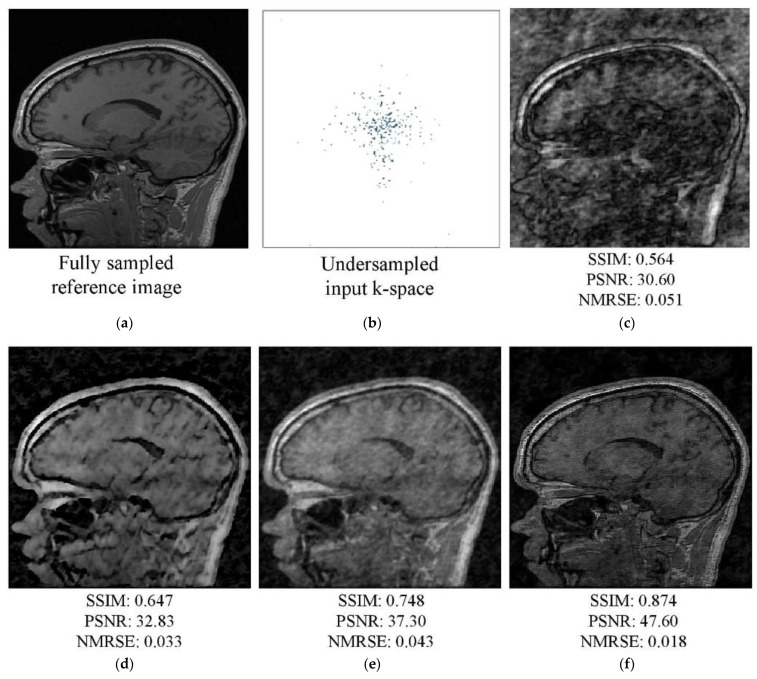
MRI images from the test dataset (slice No. 100) for AF 6: (a) fully sampled reference image and (b) undersampled k-space. Image reconstructed by the (c) IFFT, (d) Unet, (e) W-net, and (f) RA-CNN methods.
